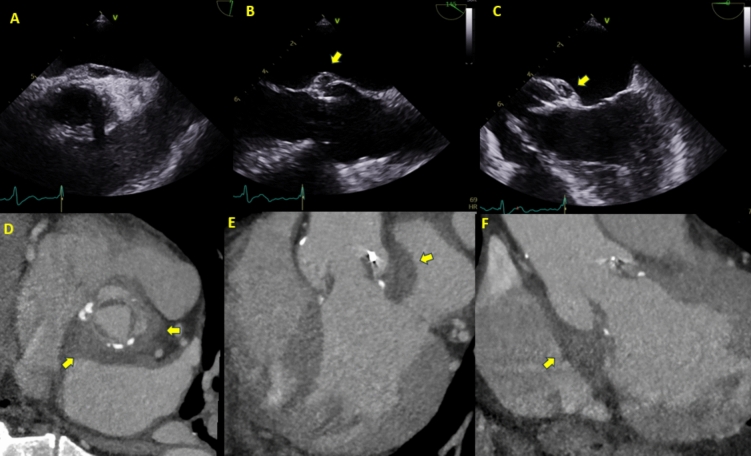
67-year-old gentleman presenting with a 4-day history of malaise, fatigue, and fevers. History of 25 mm Mosaic tissue aortic valve replacement 6 years prior to this presentation. Blood cultures were positive for *Methicillin-Sensitive Staphylococcus Aureus* (MSSA). Transthoracic echocardiogram revealed thickened valve leaflets and abnormal thickening along the posterior aortic root with an echolucent space, suspicious for an aortic root abscess. These findings were confirmed on CT coronary angiogram, which also showed significant thickening of the aortic root, particularly at the non-coronary and left coronary cusp positions. The patient was referred for surgery, during which frank pus was drained from beneath the non-coronary cusp. The *top panel* (A, B, C) shows transesophageal echocardiogram findings suspicious for an aortic root abscess with thickened aortic valve leaflets. The *bottom panel* shows CT coronary angiogram images demonstrating aortic root thickening consistent with the abscess
